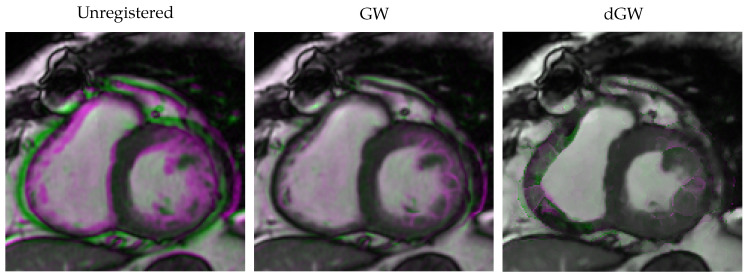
Differences between GW and dGW registration. From left to right: unregistered cine cardiac MRI, GW registration, dGW registration. Pink: registered systole with higher intensity. Green: registered diastole with higher intensity.
